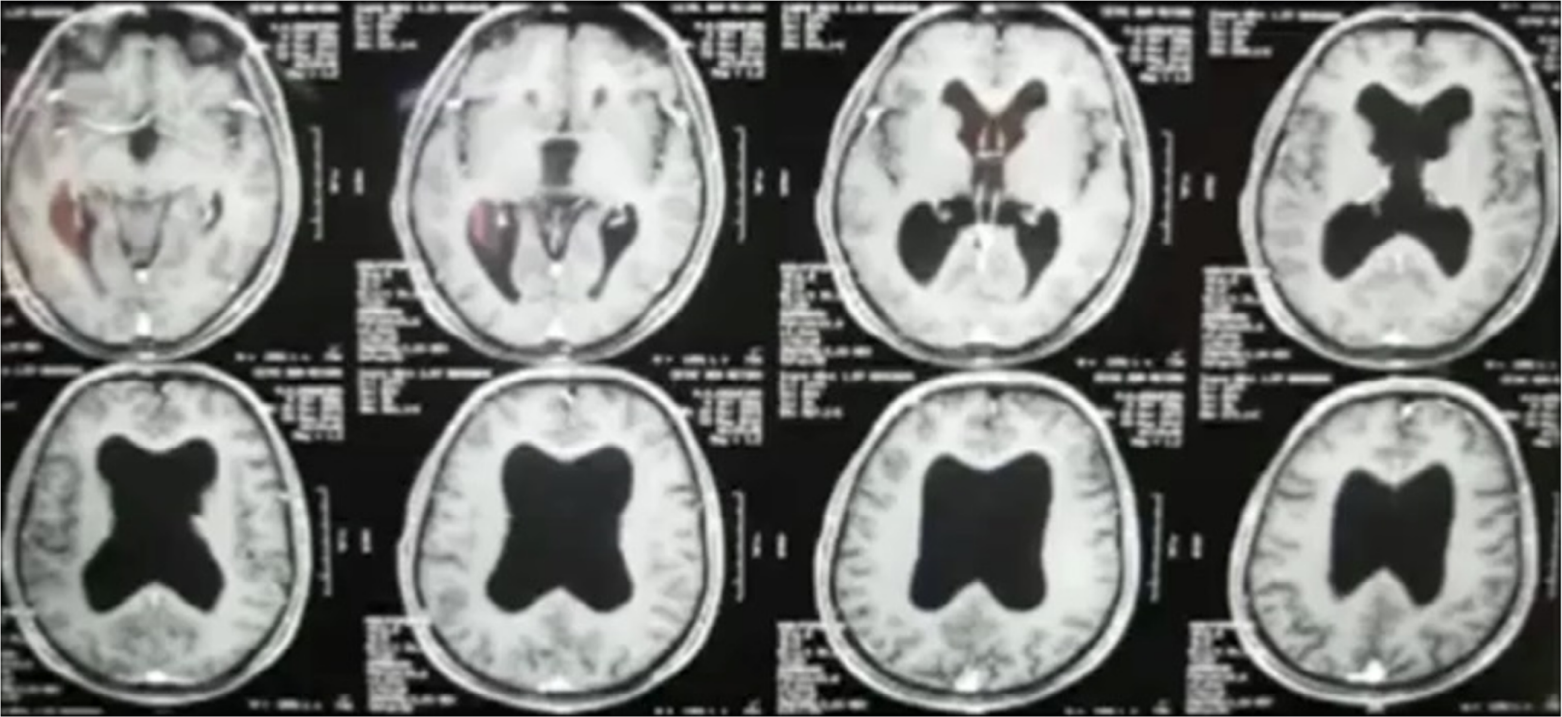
The skilled task being trained is to view a set of CT slices, and then, on the basis of reasoning about those views, to plan a point of entry on the skull and then introduce the EVD into the ventricular system.
